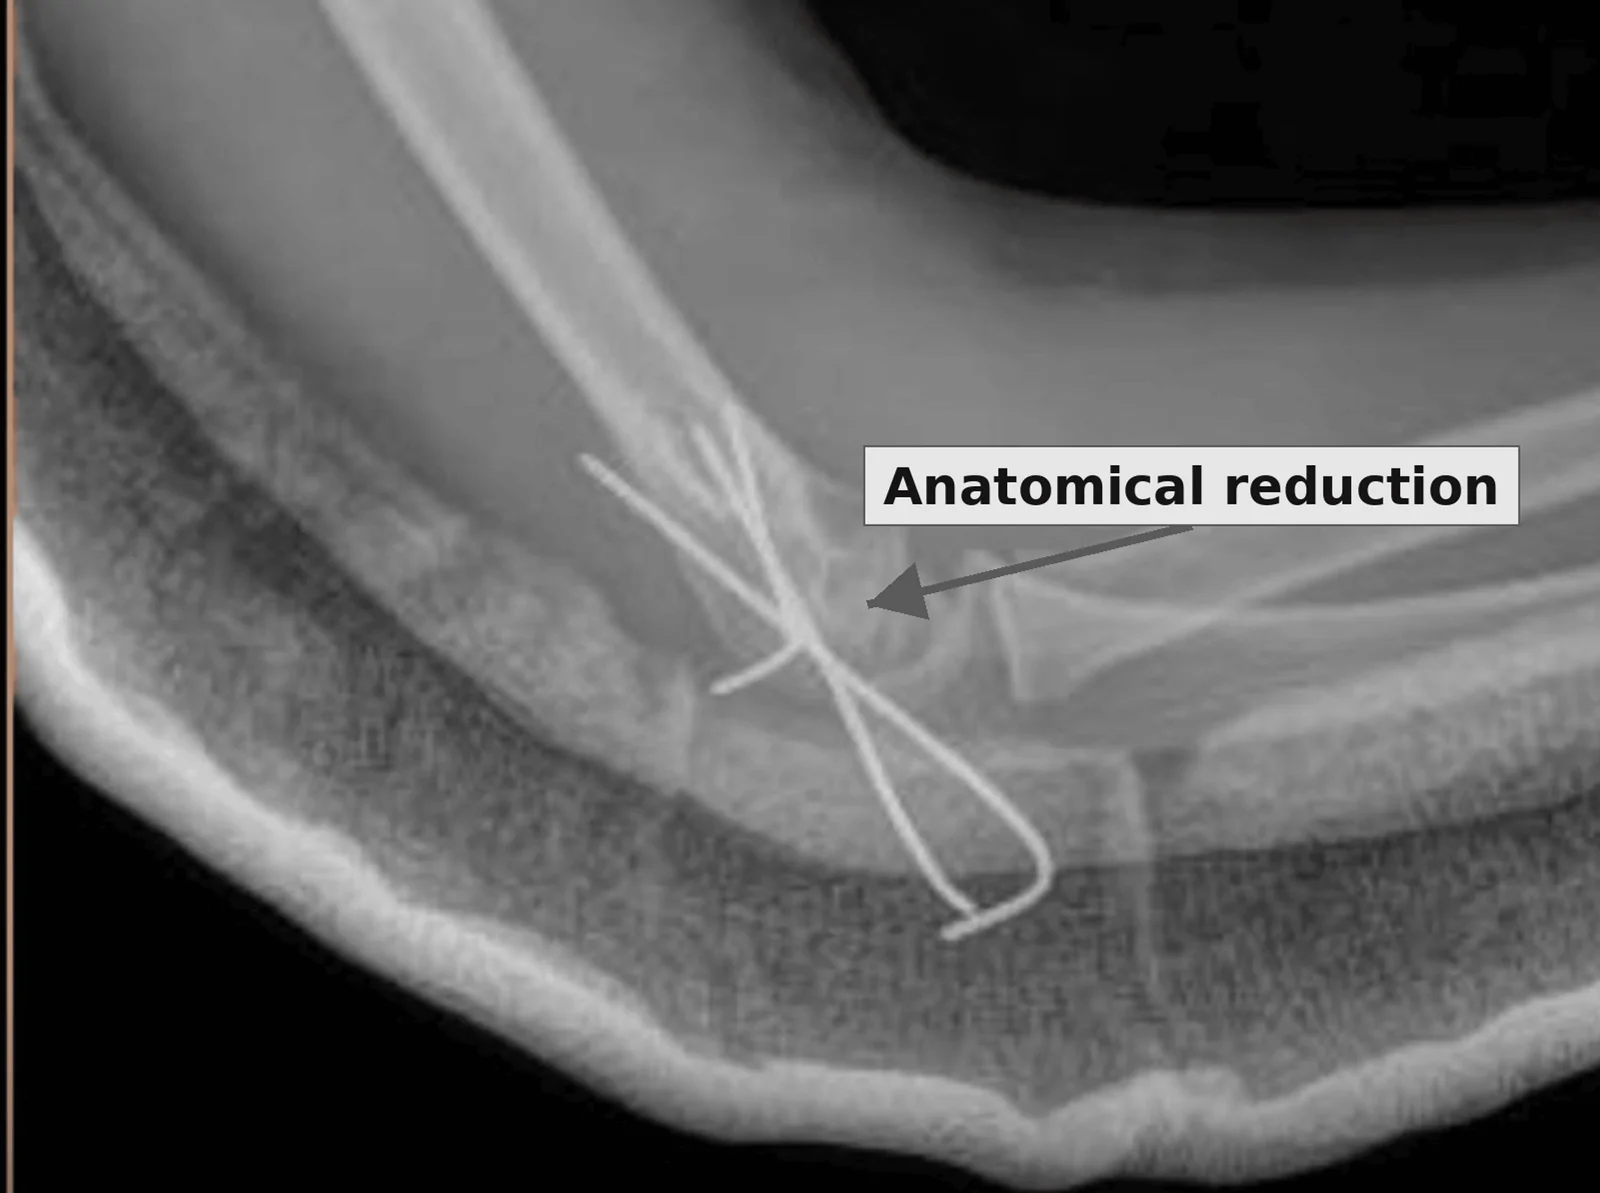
Lateral radiograph of the right elbow following open reduction and internal fixation demonstrating anatomical reduction secured with divergent 1.6 mm Kirschner wires.
